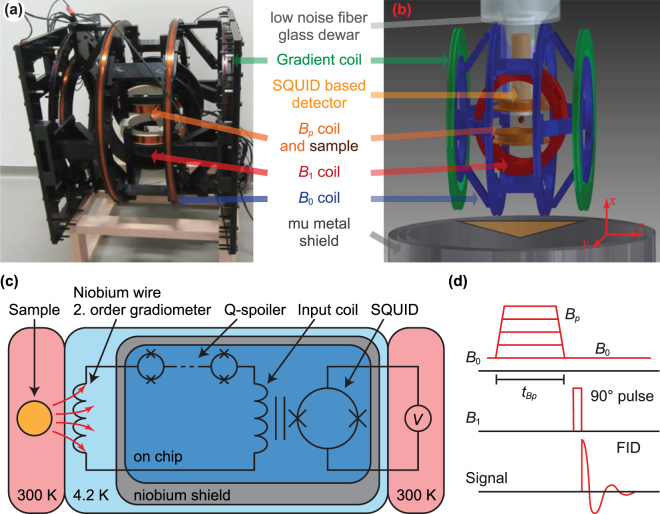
Photo (a), scheme of the ULF MRI system (b), scheme of the SQUID based magnetic field detector (c) and the pulse sequence used for the ULF NMR measurements (d).
